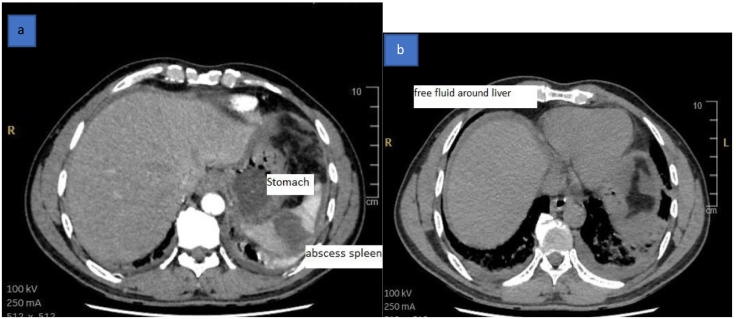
CT scan of the abdomen showing a: splenic abscess, b: free fluid around the liver.
